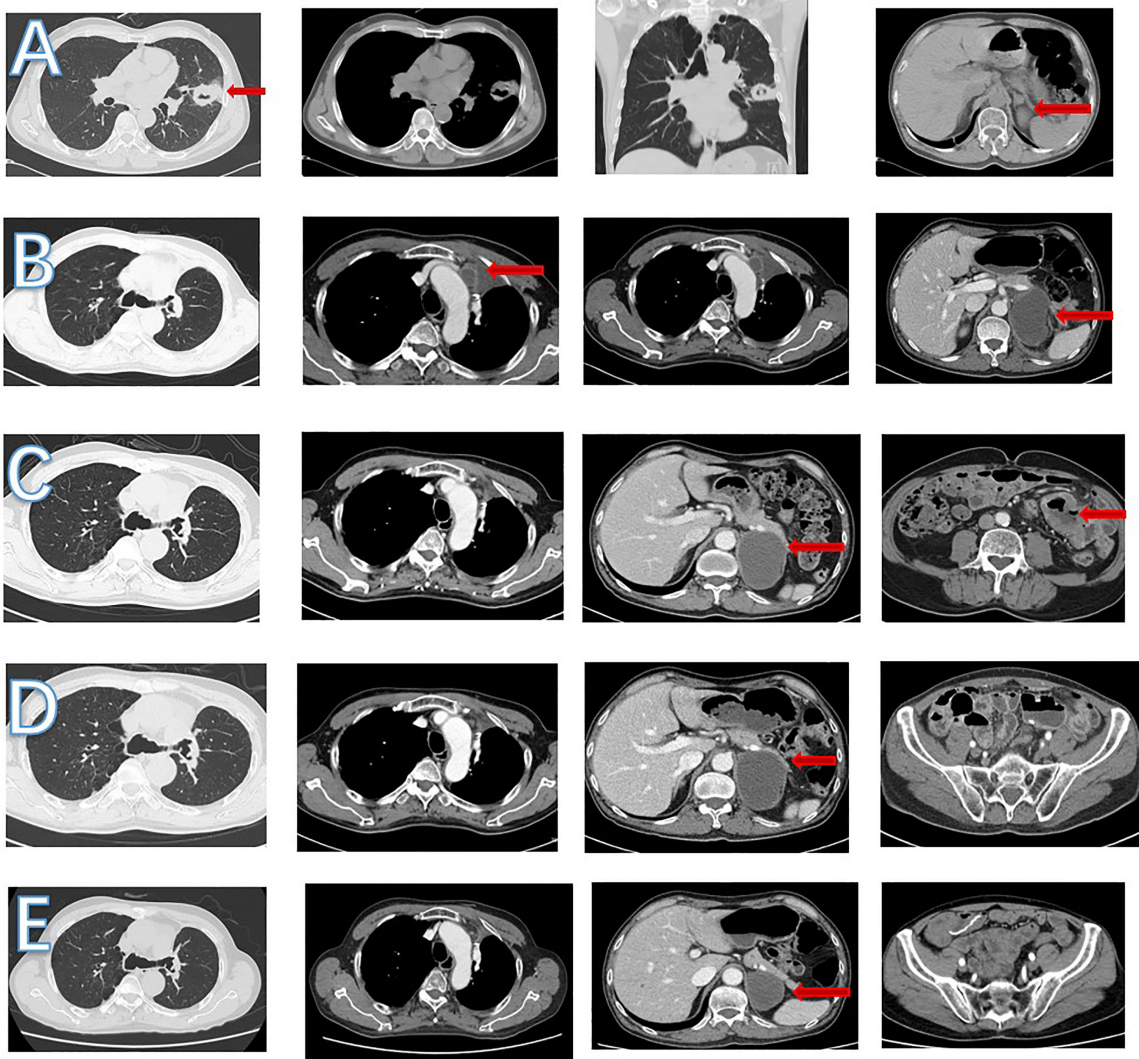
CT scan images during treatment. (A) In June 2018, left lung nodules and suspected metastasis of left adrenal nodule. (B) In September 2018, enlarged mediastinal lymph nodule and increased left adrenal nodule. (C) In April 2020, a new small bowel mass was found, left adrenal nodule was stable and mediastinal lymph nodule shrank. (D) In July 2020, left adrenal and mediastinal lymph nodule was stable. No small intestinal tumor was found. (E) In March 2022, left adrenal nodule shrank and mediastinal lymph nodule was stable.
